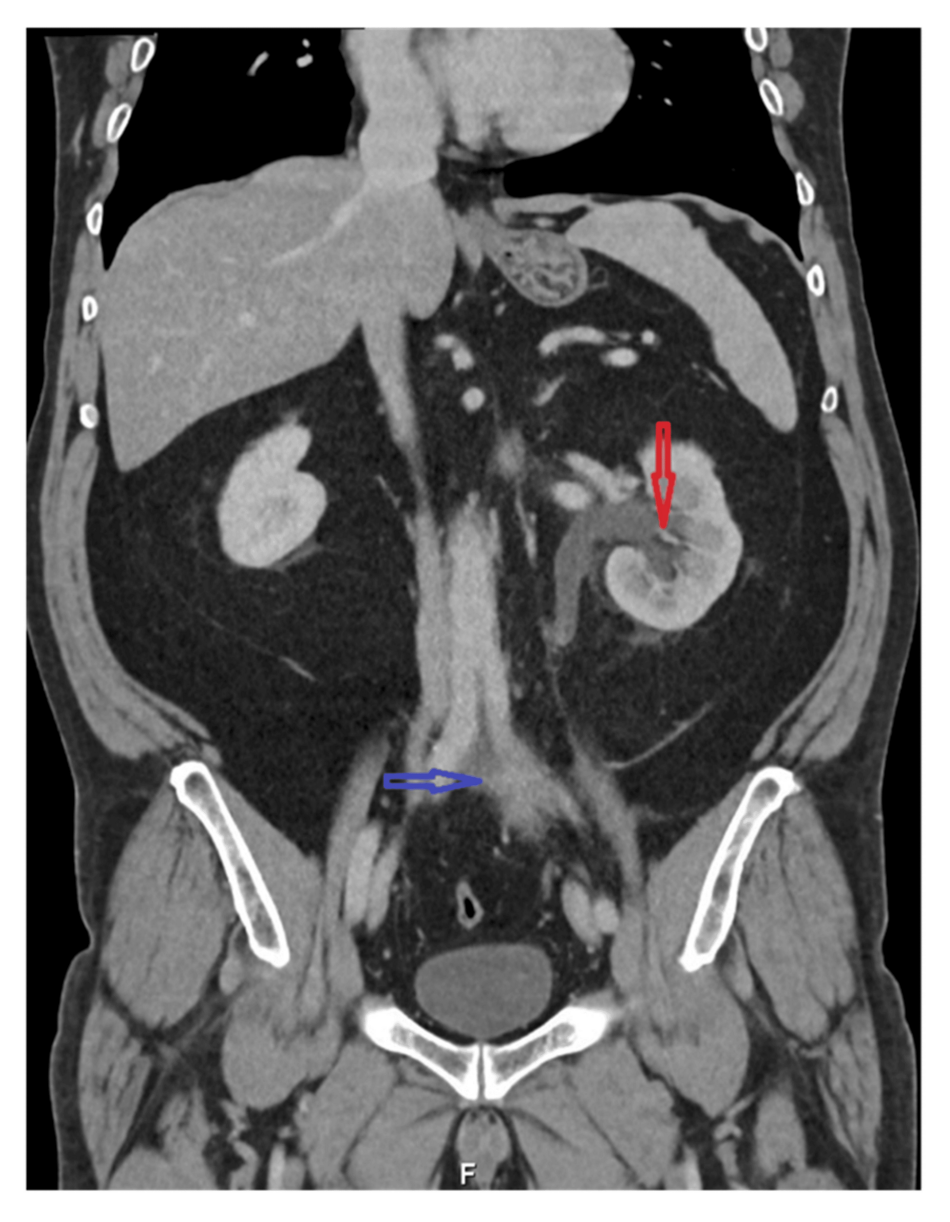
Abdominal and pelvic contrast-enhanced CT Contrast-enhanced abdominal computed tomography (coronal view) showing irregular left-sided retroperitoneal soft-tissue thickening anterior to the left common iliac vessels (blue arrow), associated with mild to moderate ipsilateral hydronephrosis (red arrow).
